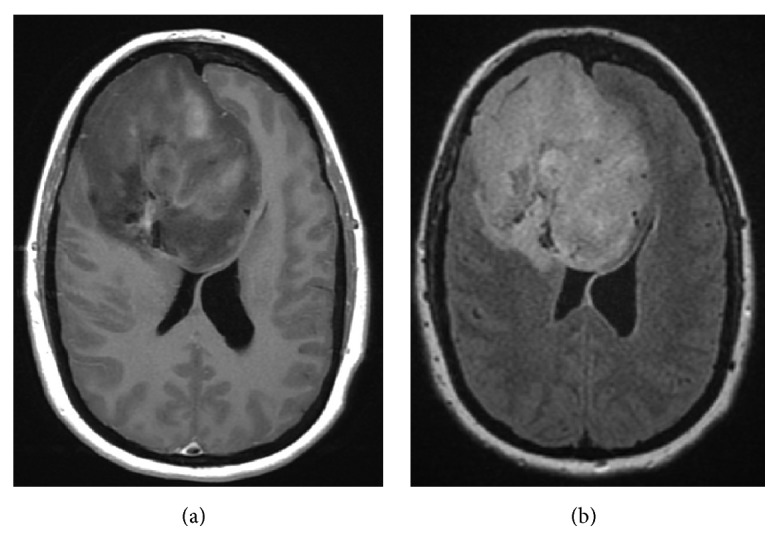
Axial MRI shows a 7.5 cm heterogenous mass in the right frontal lobe with T1-weighted sequences showing patchy enhancement following contrast administration (a) and hyperintensity with surrounding vasogenic edema on T2-weighted fluid attenuated inversion recovery sequences (b).
